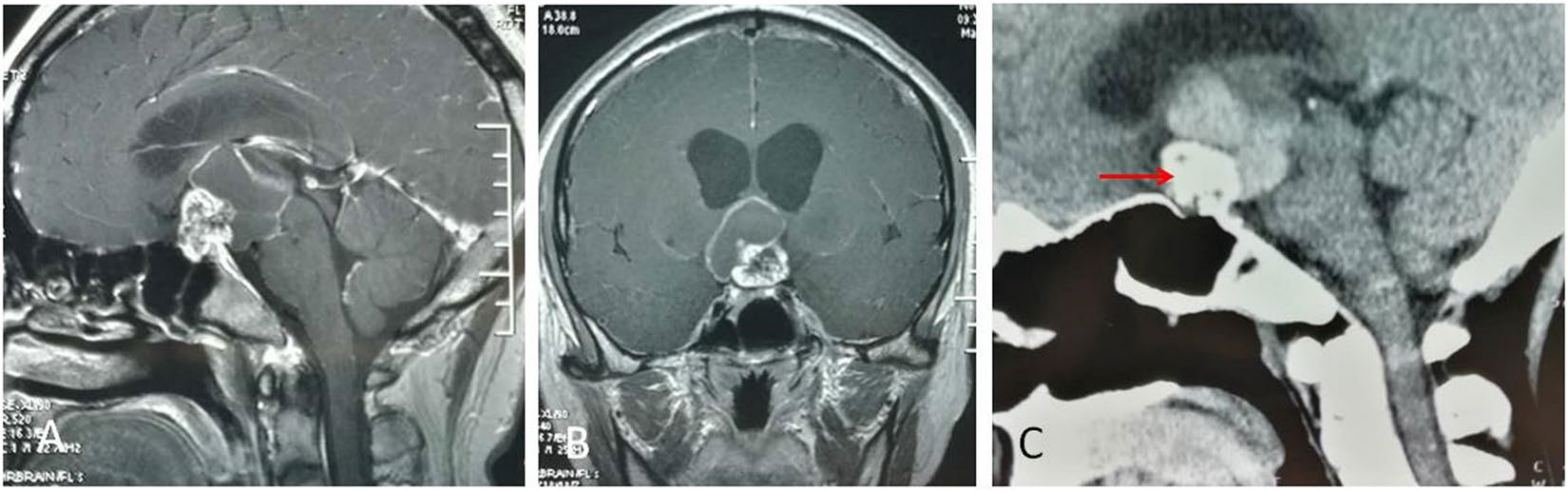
Example of a Hypothalamic stalk Cp (pathological confirmed as adamantinomatous Cp) grows in vertical direction. (A and B) Sagittal (A) and Coronal (B) postcontrast T1-weighted MR images showing that the upward growth tumo towards to the third ventricle is cystic, while the downward growth tumor towards to suprasellar cistern is solid. (C) The CT scan showing that plaque calcification can be found in the cisternal part (red arrow).
